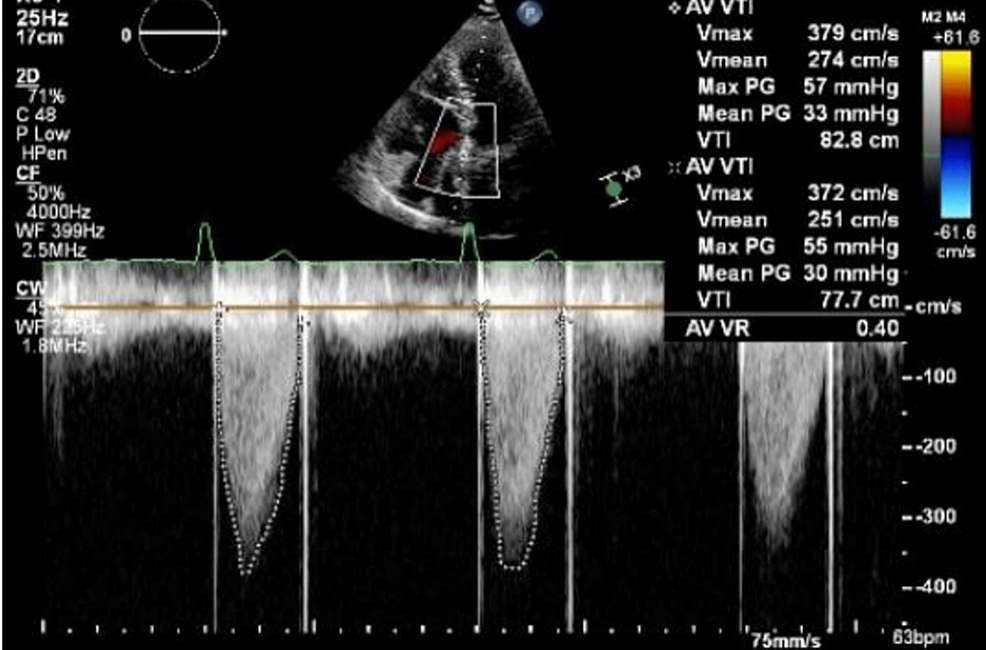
Repeat TTE post second cycle of tPA reported a mean and peak systolic gradient across the aortic valve of 39.2 and 65.1 mmHg, respectively. TTE, transthoracic echocardiogram; tPA, tissue plasminogen activator
